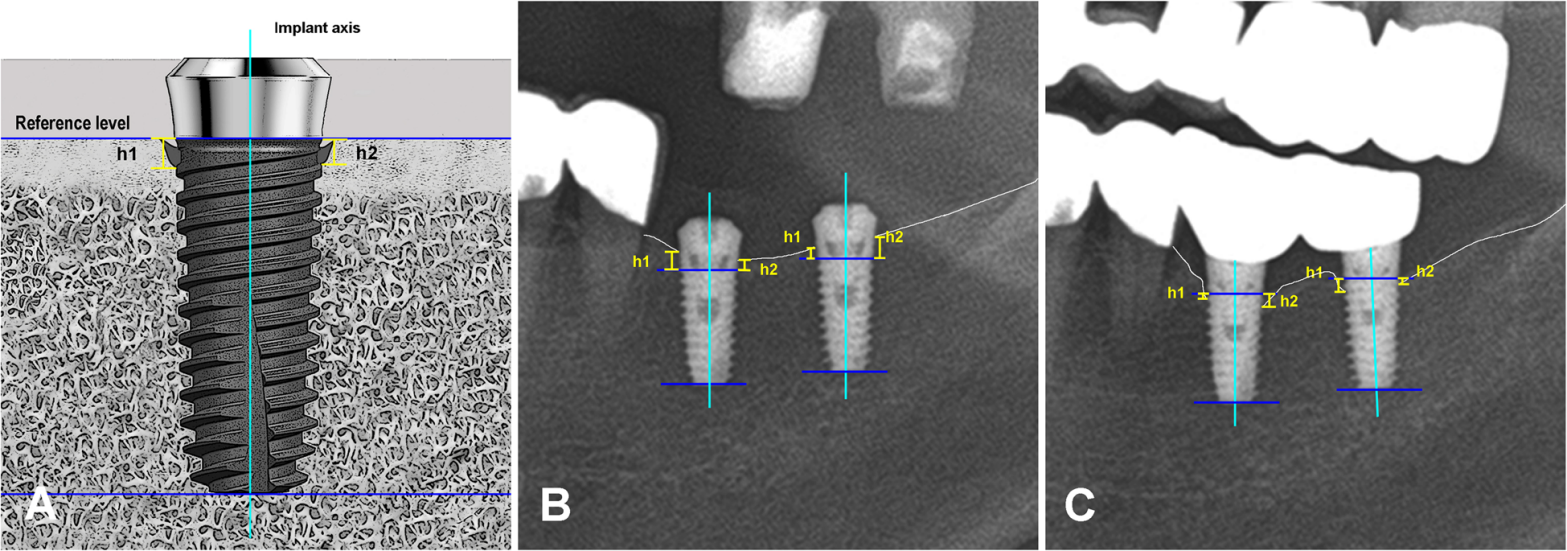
An example of the measurement of the MBL on the mesial and distal sides of the implant system (a) using panoramic radiographs taken after placement (b) and 1 year after loading (c). Marginal bone level on the mesial side, h1; marginal bone level on the distal side, h2. Both radiographs (b–c) depict the Stella® implant system 4 mm in diameter and 8.5 mm in length placed in the same patient
